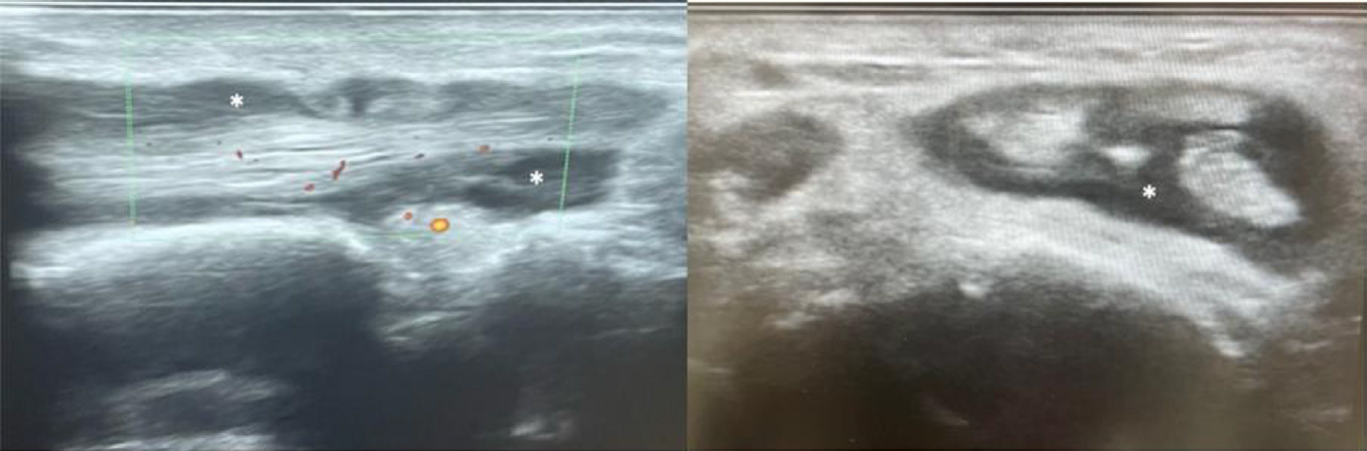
Longitudinal (left) and axial (right) ultrasound images showing tenosynovitis of the radial tendons of the right wrist (asterisk).
